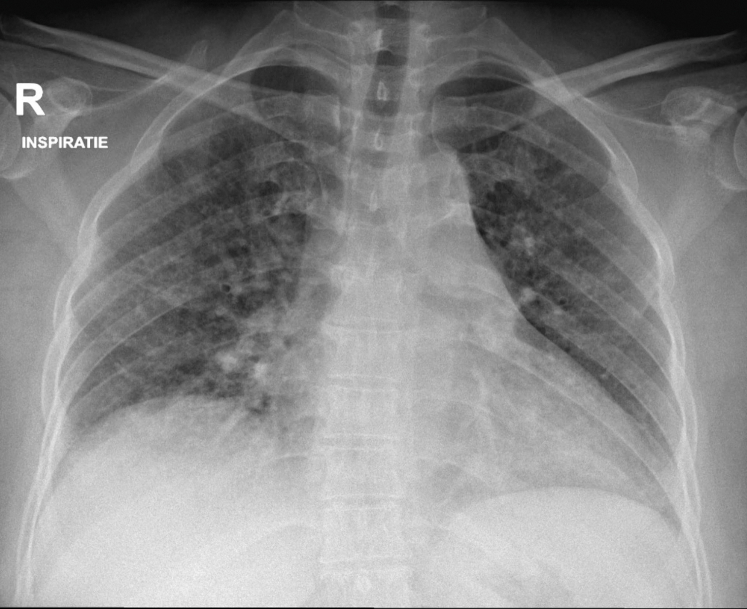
Chest radiograph on presentation.
